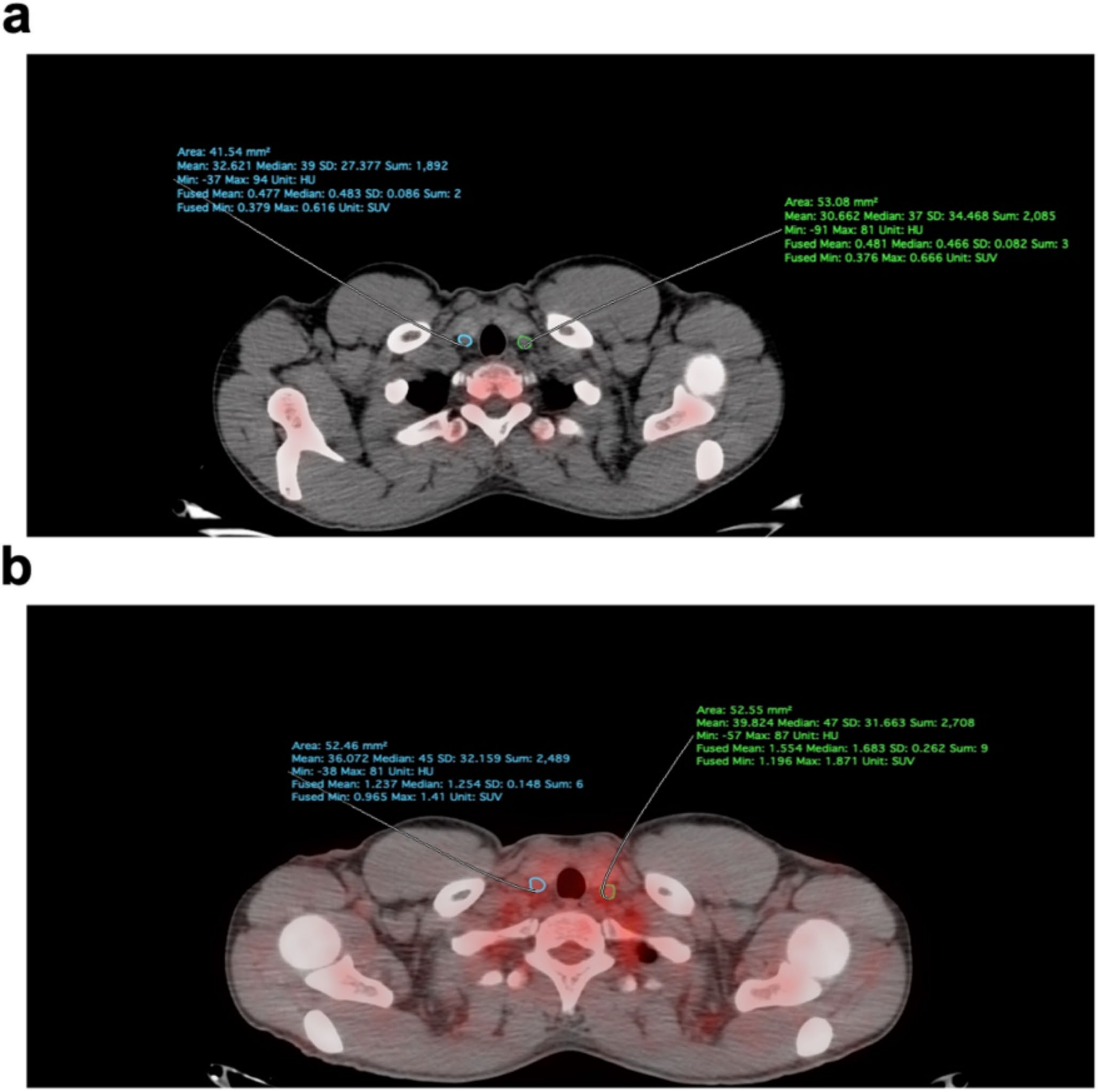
Axial PET/CT image demonstrating manually delineated regions of interest (ROI) around the LCC and RCC to obtain quantitative values (SUVmean, SUVmax) of (a) NaF and (b) FDG uptake for each artery. Images were analyzed and quantified using OsiriX 7.5.1 software (Pixmeo SARL)
